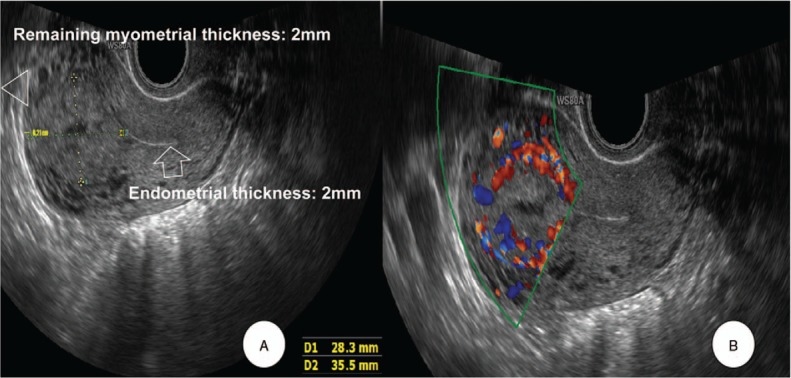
Transvaginal ultrasound. (A) Brightness-mode showed 2 mm endometrial thickness (arrow). A 3.6 × 2.9 × 2.4 cm sized heterogenous hyperechoic solid lesion with cystic vascular areas within it, was noted within the myometrium of the right fundal posterior region of the uterus, away from the endometrium. The thickness of the remaining muscle layer with invasion of the invasive mole was very thin, 2 mm (arrowhead). (B) Color Doppler showed prominent blood flow signals of various directions of arterial and venous flows within the lesion, which were also seen within the cystic areas of the lesion.
